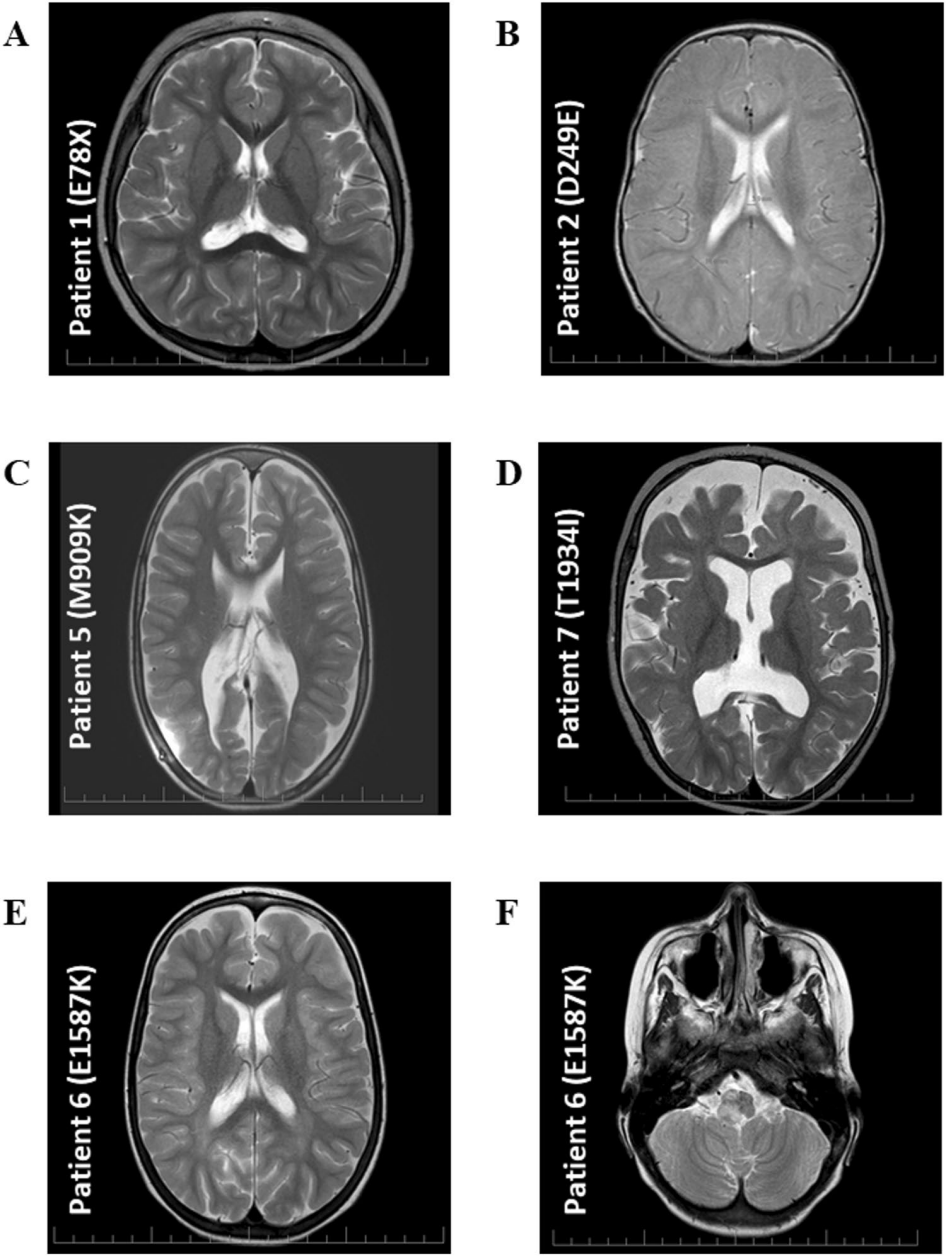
Brain MRI. (A) MRI of patient 1 at three years of age showed areal leukoencephalopathy in the occipital periventricular areas bilaterally, without acute changes. (B) MRI of patient 2 at one year of age showed areal leukoencephalopathy in the occipital periventricular areas bilaterally, without acute changes. (C) MRI of patient 5 at seven years of age showed dysgenesis of corpus callosum, hydrocephalus on V-P drainage. (D) MRI of patient 7 at one year of age showed progressive diffuse brain atrophy. (E,F) MRI of patient 6 at six years of age showed frontal and occipital areas of leukoencephalopathy, tumor in the brainstem.
